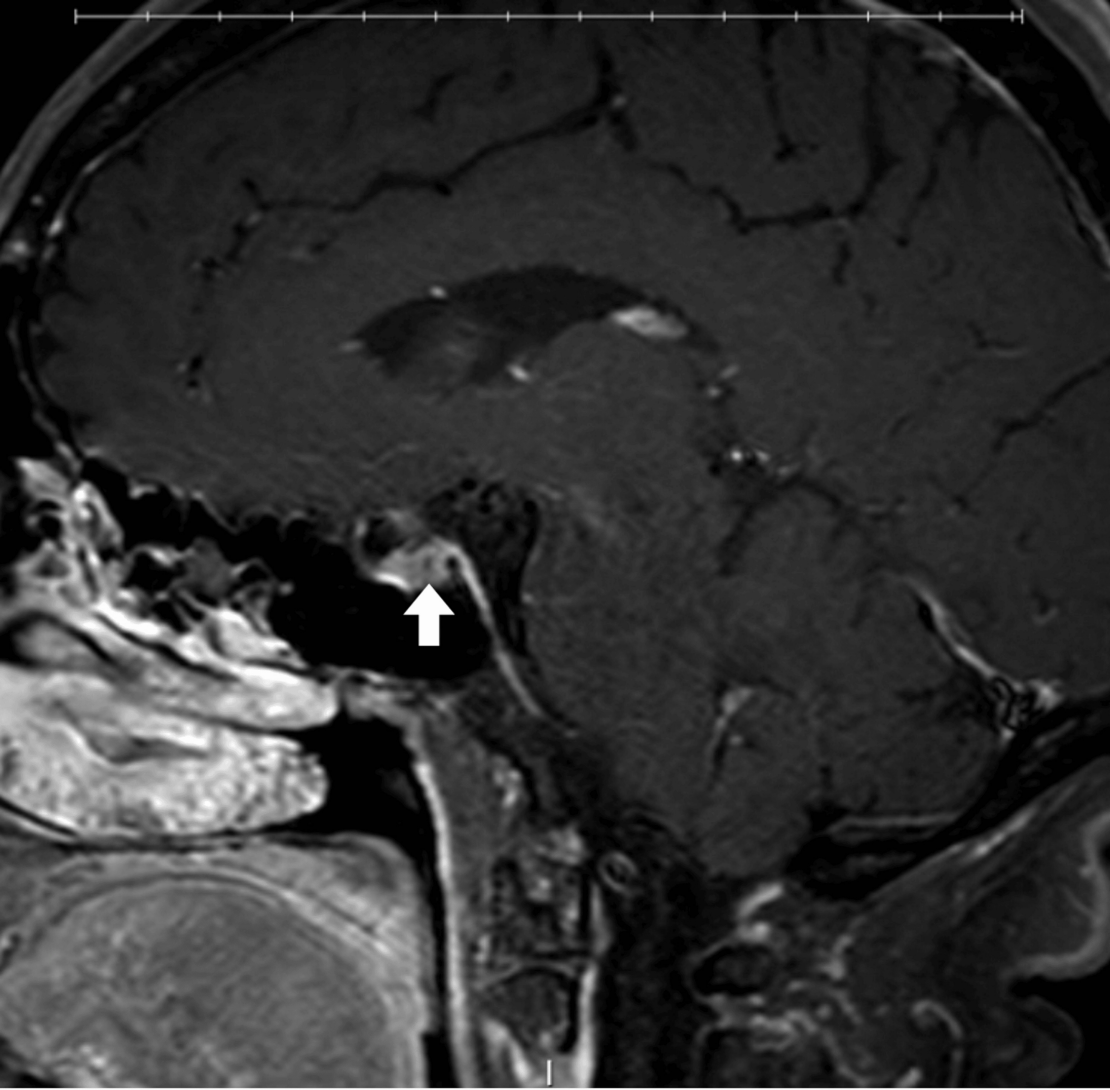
Contrast-enhanced T1-weighted fat-saturated brain MRI in the sagittal view shows a punctate focus of hypoenhancement (arrow) compared to the normally enhancing surrounding pituitary gland.
